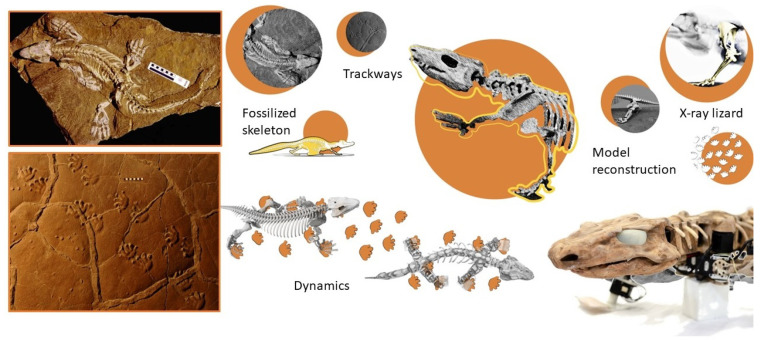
OroBOT, a moving robot model of the *Orobates pabsti* stem amniote based on CT scans of its skeletal remains and trackways. Image courtesy of Thomas Martens, Museum der Natur Gotha; Sebastian Voigt, Urweltmuseum Geoskop Thallichtenberg; Alessandro Crespi, EPFL Lausanne; John A. Nyakatura, Humboldt University, Berlin.
